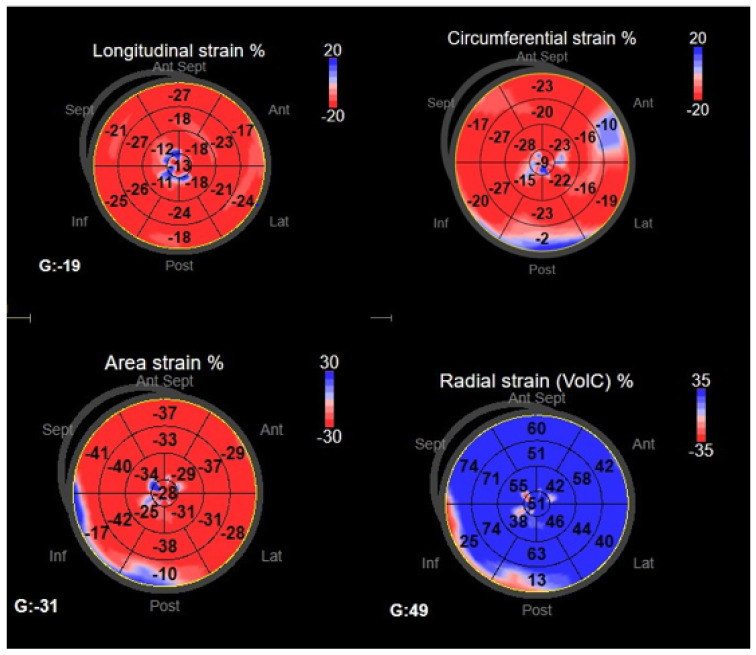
Representative four-dimensional speckle-tracking echocardiographic analysis of left ventricular myocardial deformation. The figure displays color-coded strain maps and quantitative measurements of global longitudinal strain (GLS), global circumferential strain (GCS), global radial strain (GRS), and global area strain (GAS).
